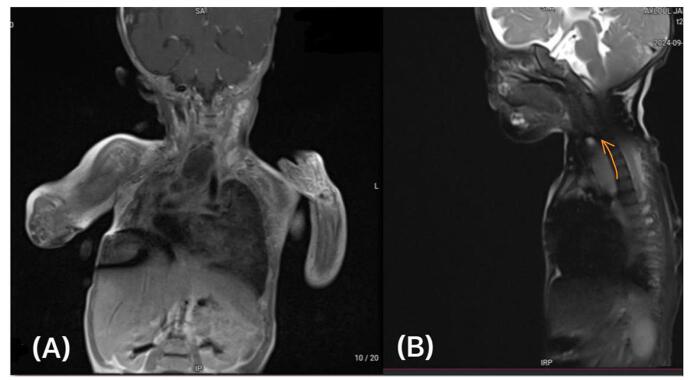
(A) Multiplanar/multisequence T1-weighted imaging of the neck and chest (without intravenous contrast) reveals a lobulated, tubular, thin-walled cystic lesion. The lesion appears hypointense on T1-weighted imaging, with no significant enhancement post-contrast. Located in the posterior mediastinum, it extends tortuously for approximately 5.4 cm from the esophageal hiatus (CS level) to the D6 level, adjacent to the anterior esophageal wall. Maximum diameters: 1.6 cm (anterior-posterior) and 1.2 cm (transverse). Findings are consistent with a large esophageal duplication cyst. (B) Lateral T1-weighted MRI of the neck shows the same large, hypointense cystic lesion (orange arrow) near the esophagus, confirming the diagnosis. The cyst has smooth margins and no signs of tissue invasion.
